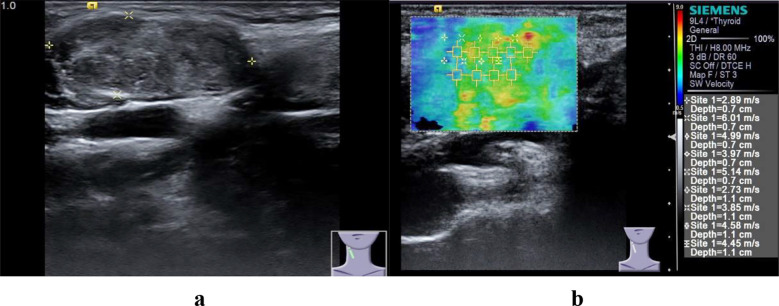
Malignant cervical LN in a 64-year-old woman. a, Conventional ultrasonic images of malignant LN. b, Elastic ultrasound image of malignant LN.
